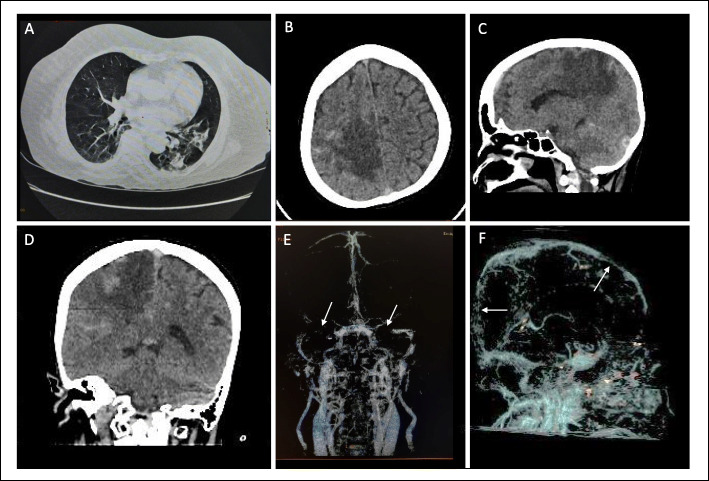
Acute onset of cerebral sinus venous thrombosis in COVID-19. A Axial thorax CT-scan showed ground-glass opacity and subpleural bilateral, with a fibrotic appearance in the left lung. B Axial head non-contrast CT-scan (NCCT scan) showed, parenchymal edema on the right parietal lobe and hyperdense cortical veins. C Sagittal NCCT scan showed parenchymal edema on the right parietal lobe and hyperdense superior sagittal sinus. D Coronal head NCCT scan showed parenchymal edema on the right parietal lobe and hyperdense superior sagittal sinus. E, F Brain CT venography showed, filling defect at SSS, TS bilateral, sigmoid sinus bilateral
